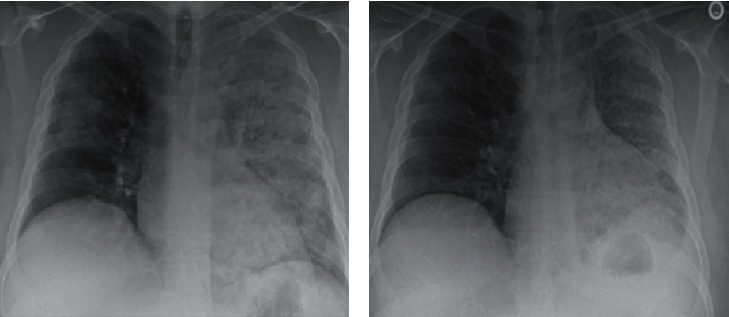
(a) Chest X-ray showing worsening left-sided infiltrates. (b) Chest X-ray showing mild improvement of left upper lobe consolidation.
